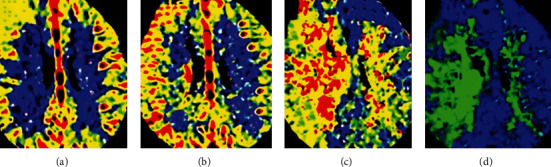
CT perfusion imaging images of patients. (a–d) disclosed the level of CBV, CBF, TTP, and MTT, respectively. White arrows pointed out the patient's focal area.
